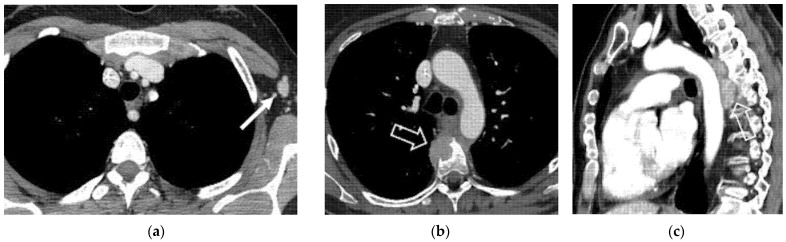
(a) A 40-year-old patient who underwent radical hysterectomy with BSO and chemoradiation therapy for cervical cancer. Axial post-contrast CT image shows left axillary lymph nodal relapse (solid arrow). (b,c) A 44-year-old patient who underwent radical hysterectomy with BSO and chemoradiation therapy for cervical cancer. (b) Axial and (c) sagittal post-contrast CT images show pathological mediastinal soft tissue infiltrating the vertebral soma (open arrows).
